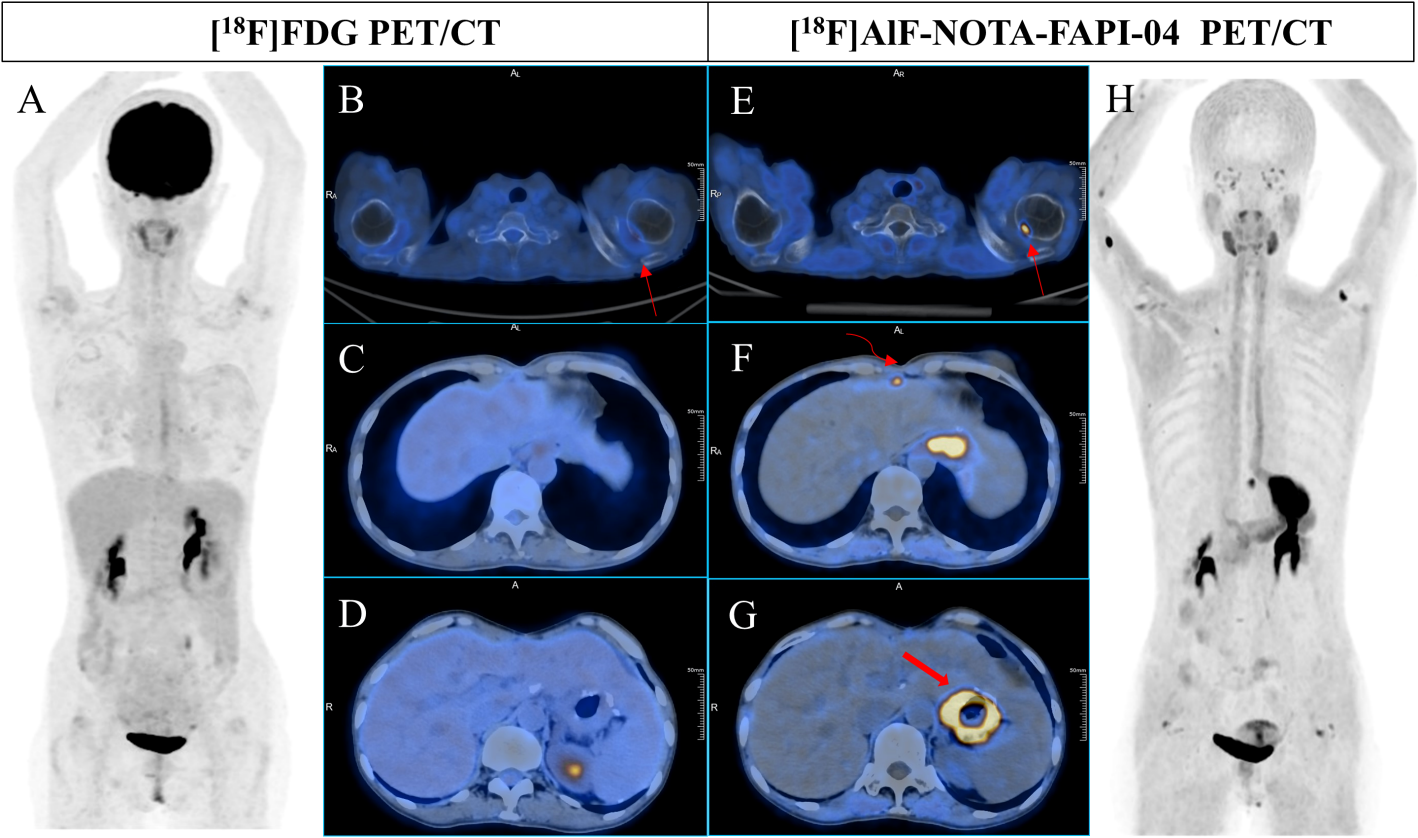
A 51-year-old woman underwent radical resection for poorly differentiated gastric adenocarcinoma thirteen months prior, followed by 4 cycles of FOLFIRI + cetuximab and 4 cycles of XELOX + cetuximab postoperative therapy. During routine follow-up, gastric endoscopy confirmed anastomotic recurrence. Subsequent [^18^F]FDG PET/CT (A-D) scans showed no abnormal uptake in the entire body. However, [^18^F]AlF-NOTA-FAPI-04 PET/CT (E-H) revealed increased tracer uptake in the right internal mammary lymph node (F, curved arrow, SUVmax 8.5) and thickening at the site of anastomosis (F, G, bold arrow, SUVmax 15.8). Additionally, [^18^F]AlF-NOTA-FAPI-04 PET/CT (E, thin arrow, SUVmax 10.9) indicated significantly high uptake at the inner edge of the right humeral head, while [18F]FDG PET/CT showed only mild uptake (B, curved arrow, SUVmax 3.4), consistent with the degenerative bone disease observed in previous contrast-enhanced CT images.
